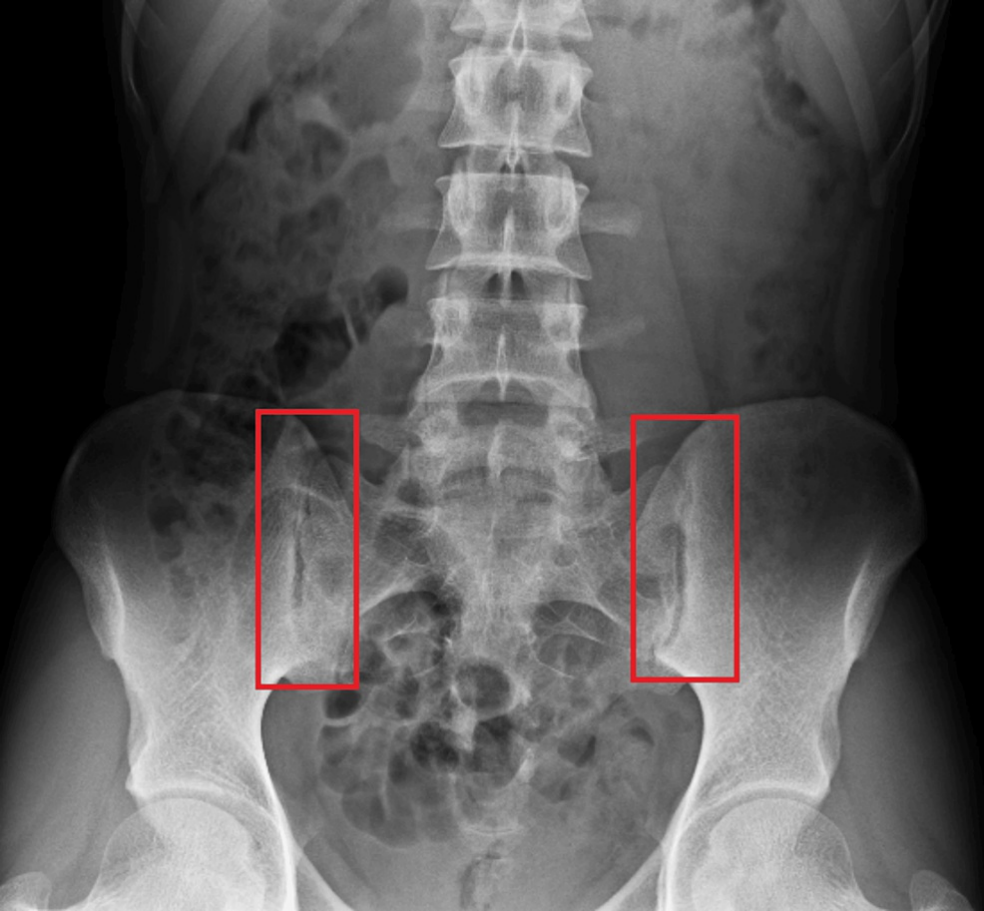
X-ray of the lumbosacral spine in the AP view showing SIJD AP: anteroposterior, SIJD: sacroiliac joint dysfunction
